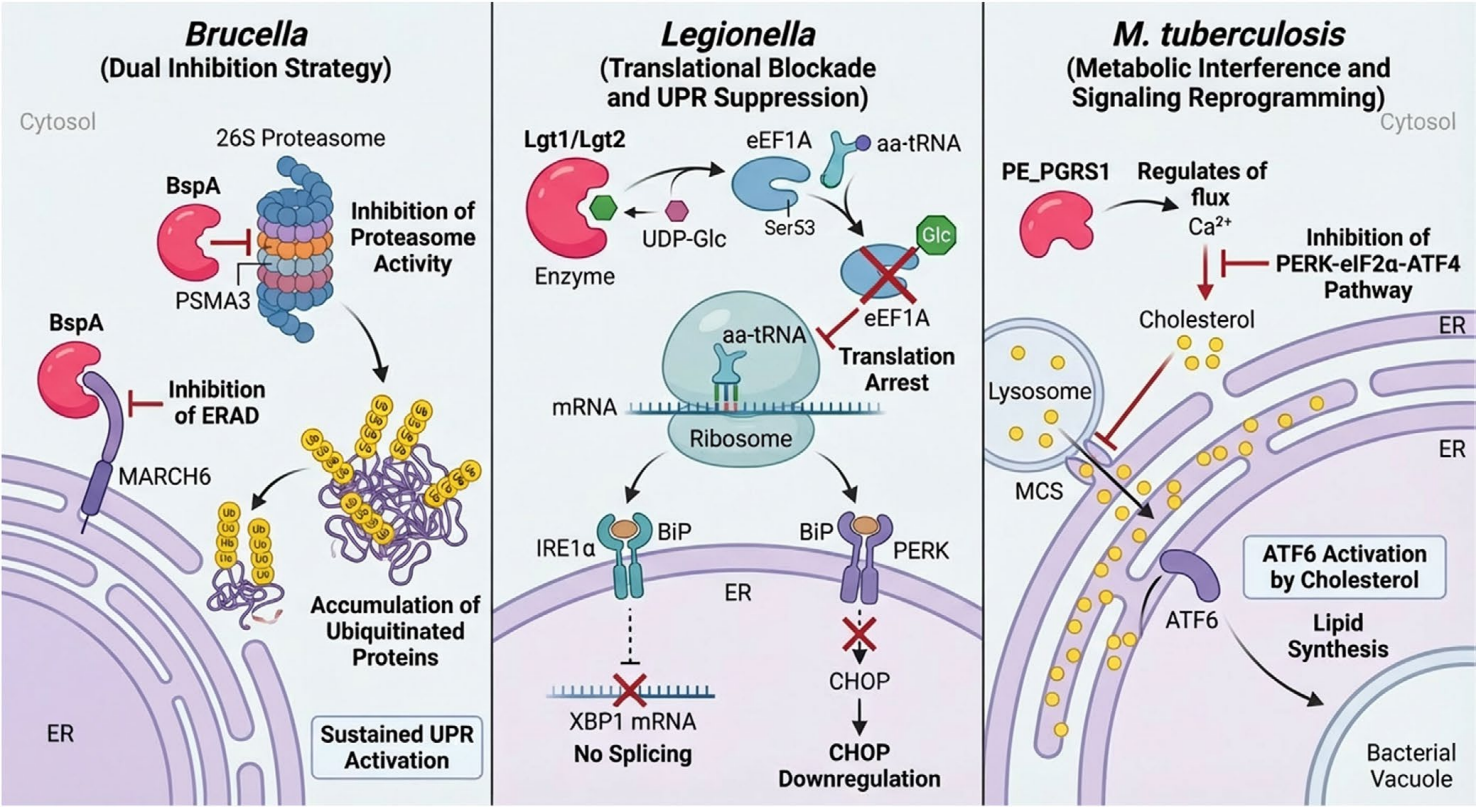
Bacterial inhibition of the unfolded protein response (UPR) during infection. (Left) *Brucella* effector BspA inhibits ERAD by targeting the E3 ligase MARCH6 and blocks proteasome activity via PSMA3, sustaining UPR activation to protect bacterial effectors. (Middle) *Legionella* effectors Lgt1/2 glucosylate host eEF1A, arresting translation and preventing the induction of IRE1α and PERK pathways. (Right)
